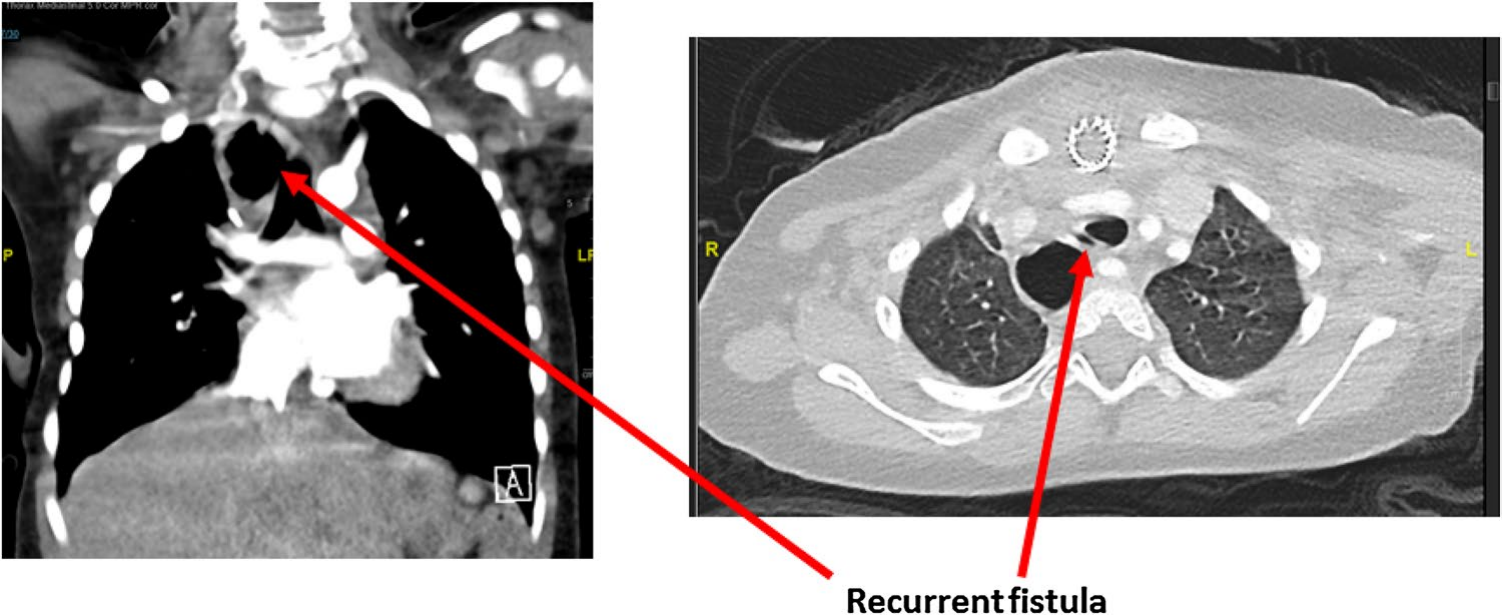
A CT scan demonstrating a complex cervical tracheo-oesophageal fistula in a patient with previous jejunal interposition—background of OA/TOF, duodenal atresia
